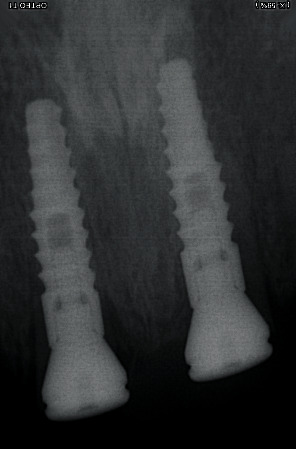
Postoperative periapical X-ray, taken immediately after implant placement.
